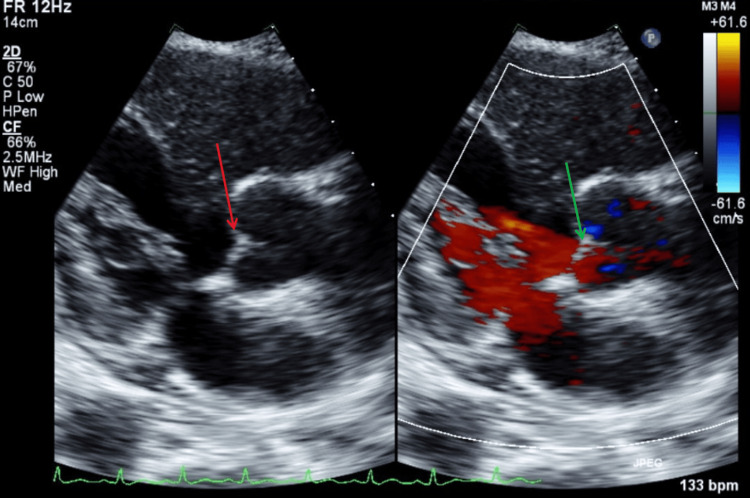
TTE parasternal long-axis view with and without color Doppler during diastole. The red arrow shows aortic valve without vegetations. The green arrow shows no signs of aortic valve regurgitation with color Doppler. TTE: transthoracic echocardiography
